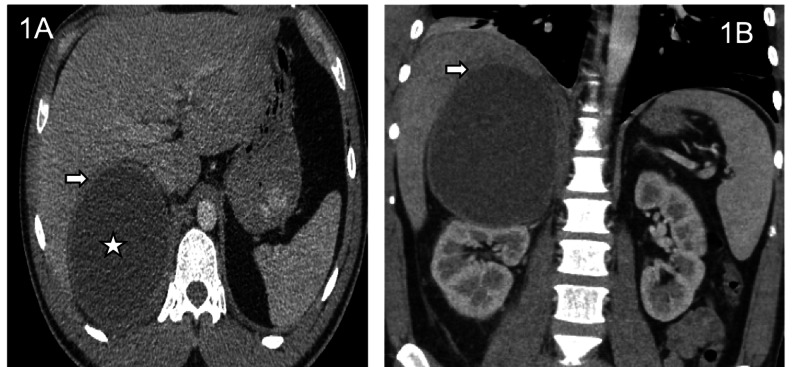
Axial and Coronal Post-Contrast CT Venous Phase Axial (1A) and coronal (1B) post-contrast CT venous phase demonstrate a large low attenuating cystic mass in the right perinephric space in the location of the right adrenal gland (star). Notice the mass effect on the right hepatic lobe (arrows). CT: computed tomography
